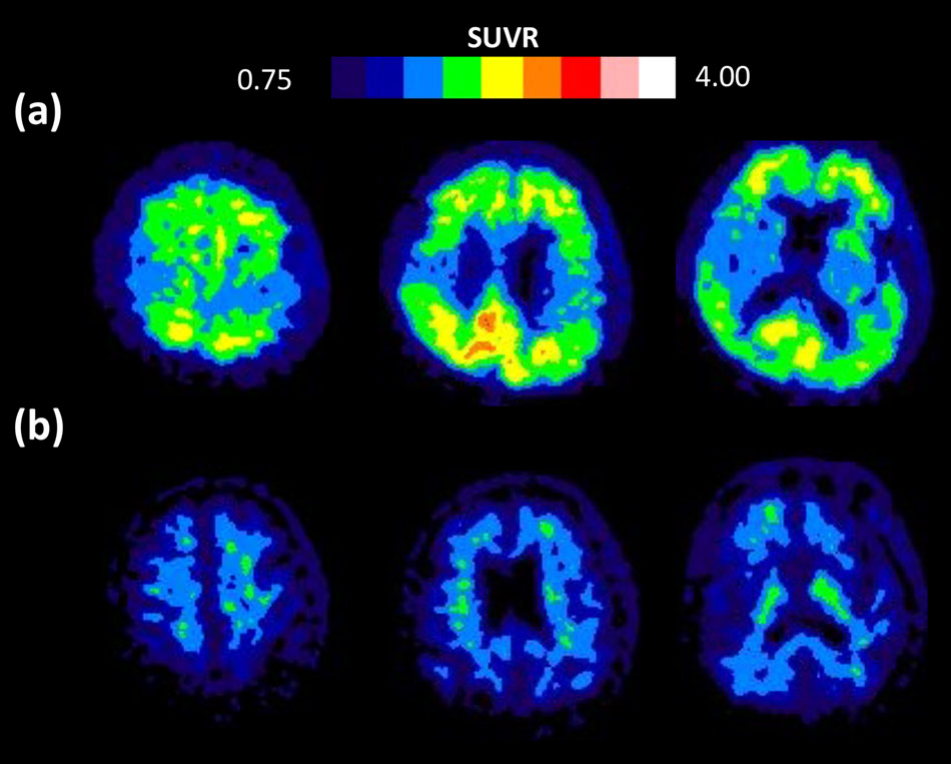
Representative positive and negative Pittsburgh compound B (PiB) scans. We defined amyloid scan positivity based on the global PiB uptake standardized uptake value ratio (SUVR) using the cutoff value of 1.2: (a) example of a positive PiB scan with a global SUVR of 1.9 and (b) a negative PiB scan with a global SUVR of 0.9.
